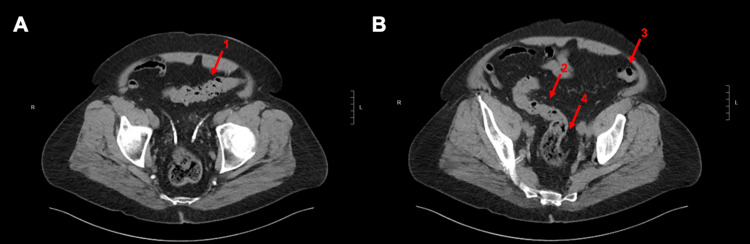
Preoperative computed tomography (CT) scan of the abdomen and pelvis without contrast five months prior to procedure (Panel A, B). Axial views demonstrating colonic diverticulosis involving the sigmoid colon (1, 2, 3) with evidence of contained microperforation. Segmental wall thickening was noted in the sigmoid colon without free fluid or abscess formation (4). The final diagnostic impression was acute diverticulitis affecting the sigmoid colon
